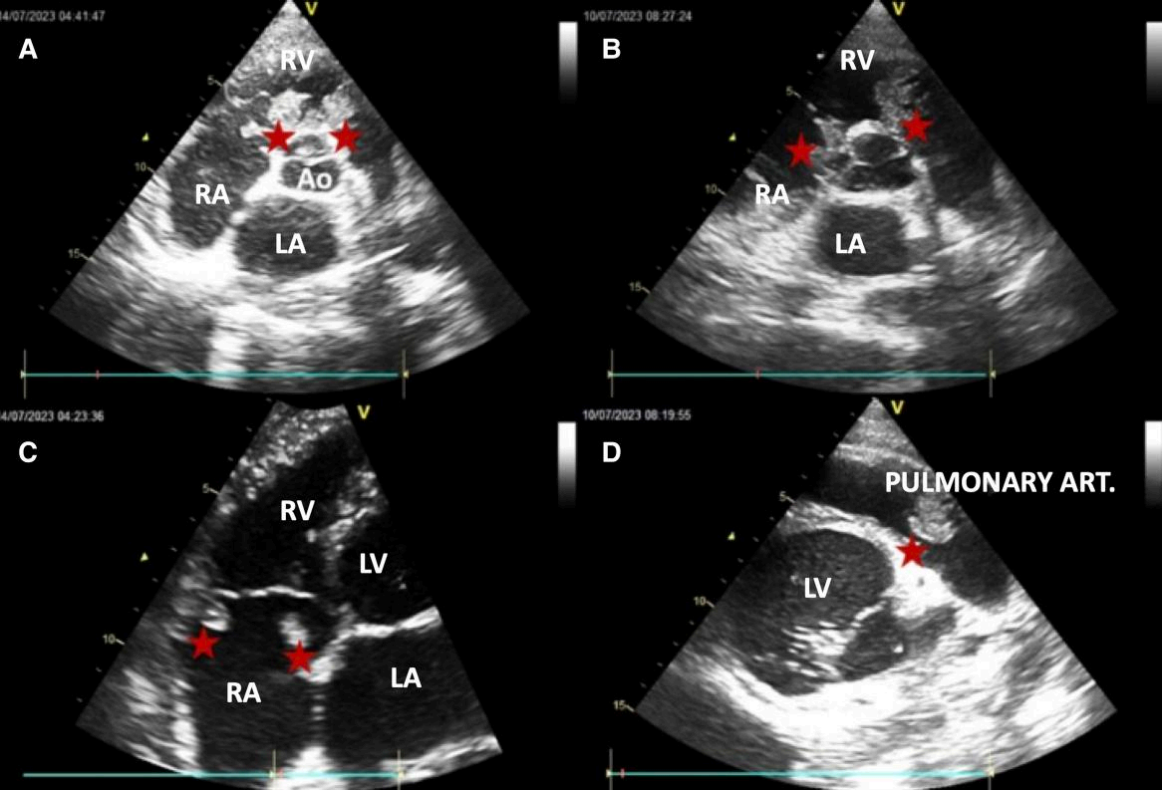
Echocardiographic sections showing thrombi. (*A* and *B*) Short-axis parasternal section passing through the root of the great vessels. (*C*) Apical four cavities centred on the RV. (*D*) Modified short-axis parasternal section centred on the trunk of the pulmonary artery. Red stars, thrombi; RA, right atrium; LA, left atrium; RV, right ventricle; LV, left ventricle; Ao, aorta.
